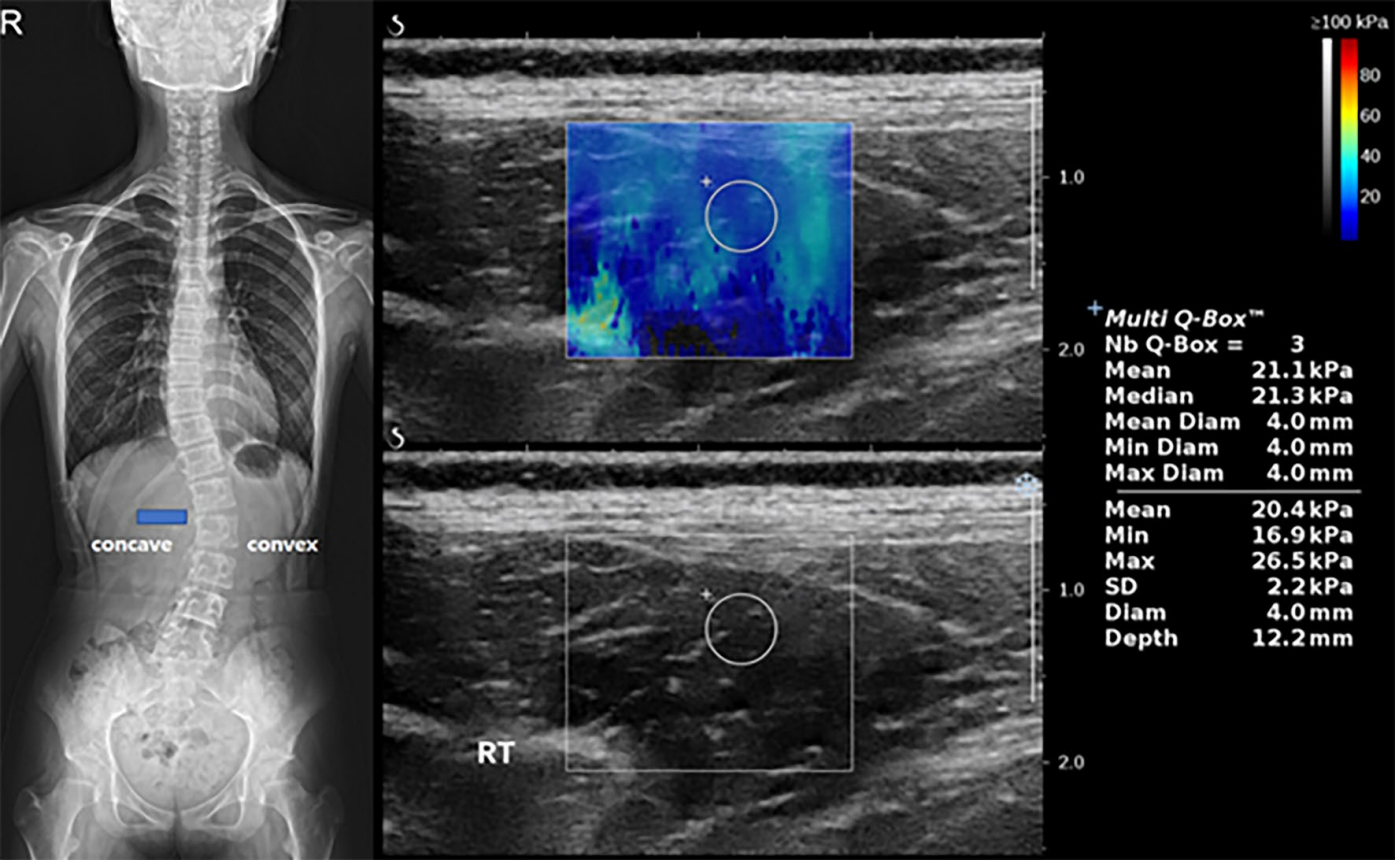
A representative case of shear wave elastography (SWE) measurement in a 12-year-old girl with adolescent idiopathic scoliosis. (A) The most curved level was determined using the standing whole spine plain film of the anteroposterior view, and it was L1/2. The blue box shows the location of the ultrasound probe when measuring SWE in the paraspinal muscle of the concave side. (B) The SWE image was obtained on the right side (the concave side of this patient) of the paraspinal muscle at the apex of the curve using a high-frequency linear probe. A round region of interest was drawn and the mean value of SWE was obtained (21.1 kPa in this image).
